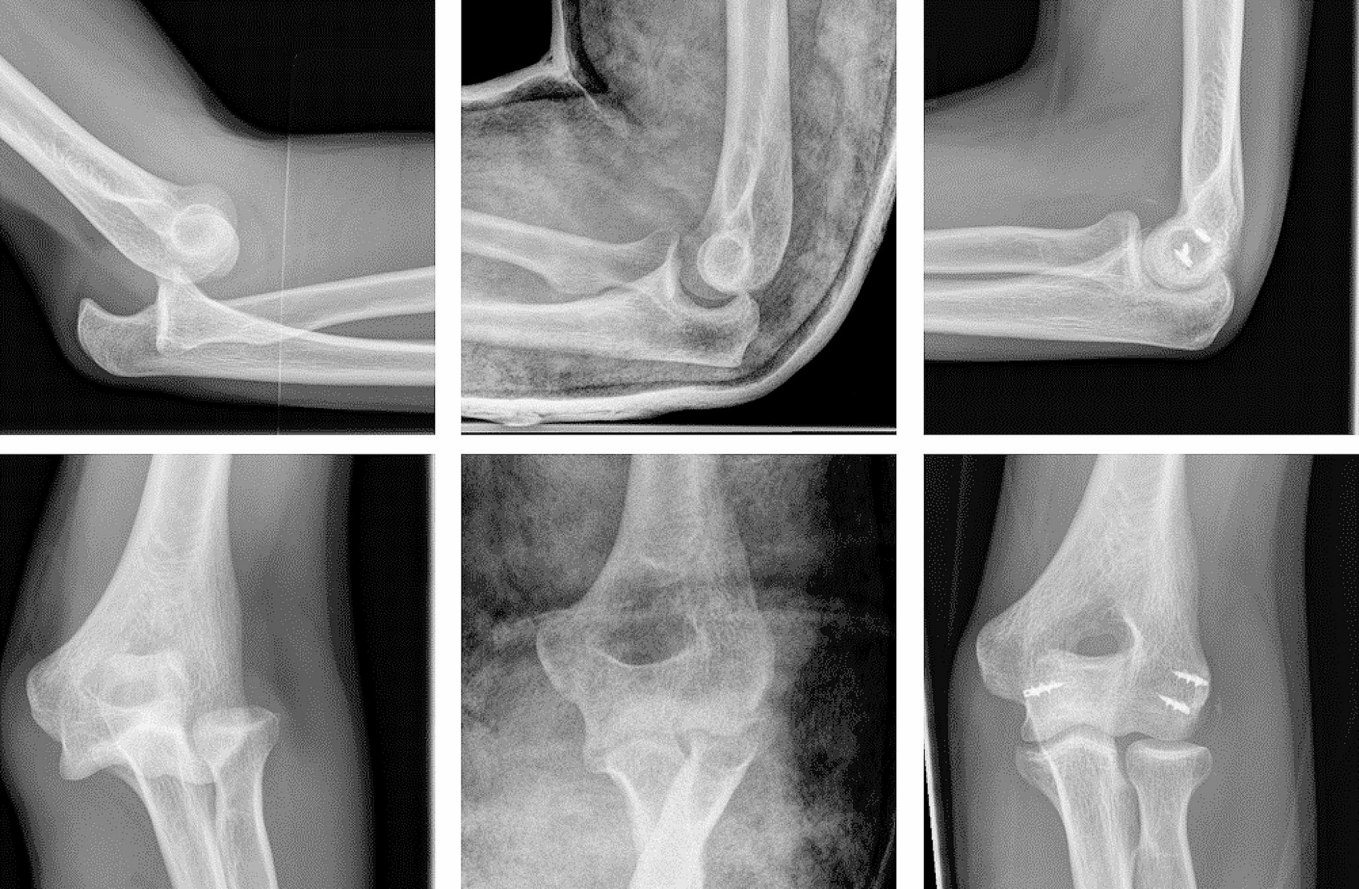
x-ray diagnostics: left acute posterior elbow dislocation after fall in bouldering; middle: drop sign indicating persisting instability after reduction; right: postoperative results after capsular-ligament reconstruction of the radial and ulnar collateral ligament and the common extensor origin
